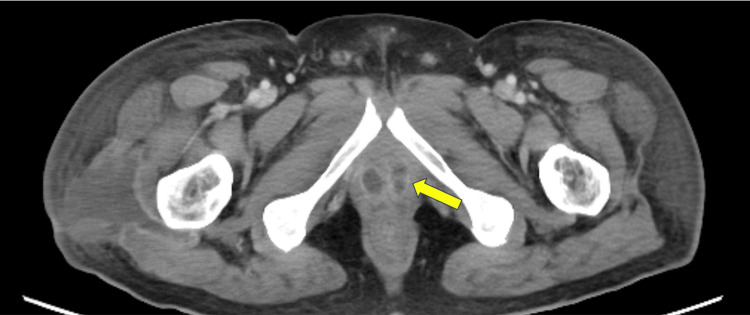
CT Imaging of a Multiloculated Prostatic Abscess CT shows a multiloculated prostatic abscess (arrow) with rim enhancement and central hypodensity, measuring approximately 4.2 × 3.8 cm.
